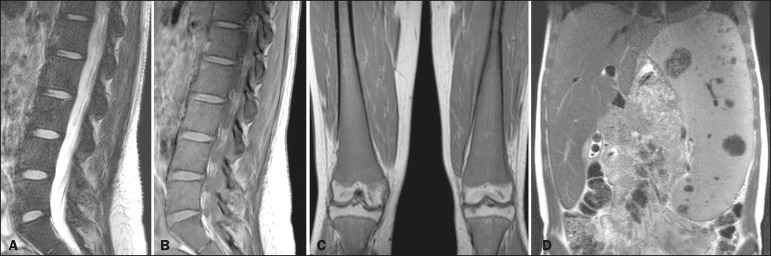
MRI of a 28-year-old woman with GD, showing extensive bone involvement with a high BMB score, together with splenomegaly (splenic volume of 2,323 cm^3)^. Sagittal T2-weighted (A) and T1-weighted (B) images of the lumbar spine, showing lower signal intensity in bone marrow than in the disc and presacral fat. After suppression of the fat surrounding the basivertebral veins, the signal intensity and extent scores were 4 and 3, respectively, resulting in an L-BMB score of 7. C: Coronal T1-weighted image of both femurs, which were scored 3 for signal intensity and 3 for the extent of involvement, infiltration of distal epiphyses being detected, resulting in an F-BMB score of 6. D: Coronal T2-weighted image of the abdomen, demonstrating massive splenomegaly, with multiple splenic infarcts.
